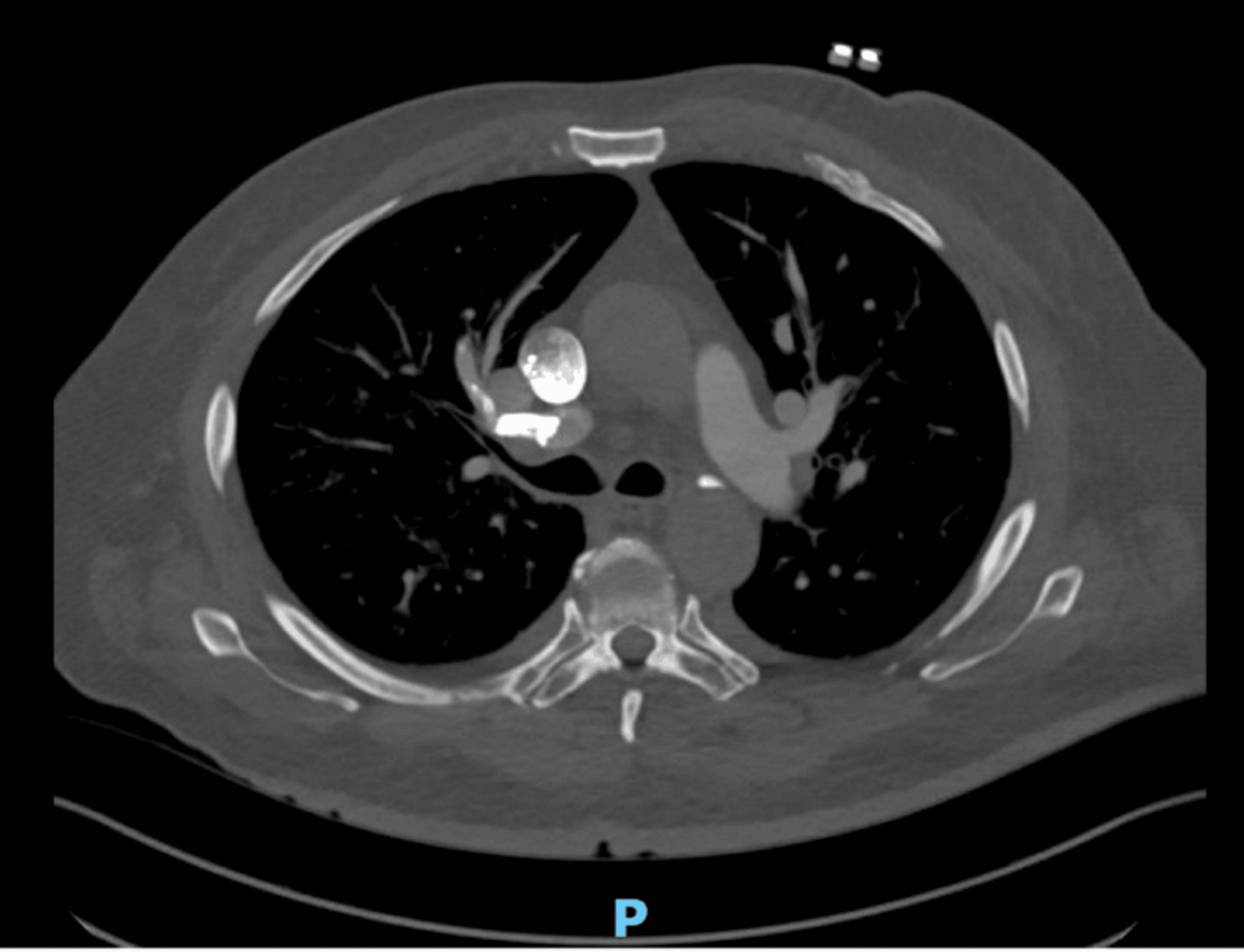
Axial CT demonstrating a hyperdense cement embolism in the right upper lobe pulmonary artery CT, computed tomography
